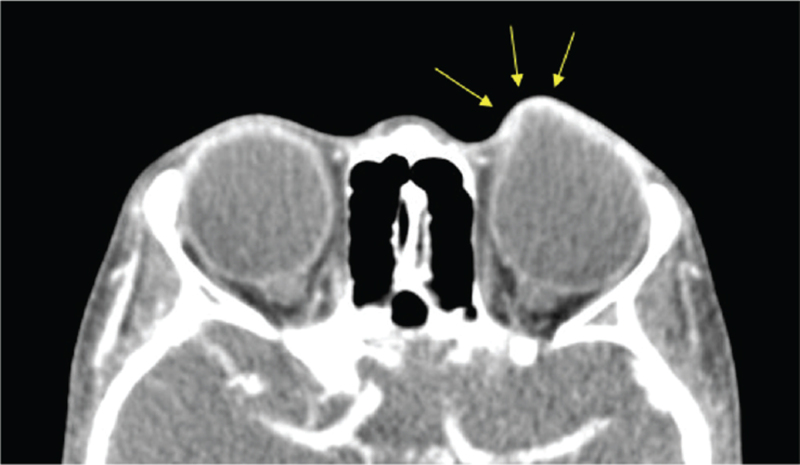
Facial computed tomography showing conical protrusion of the intercalary staphyloma (arrow).
